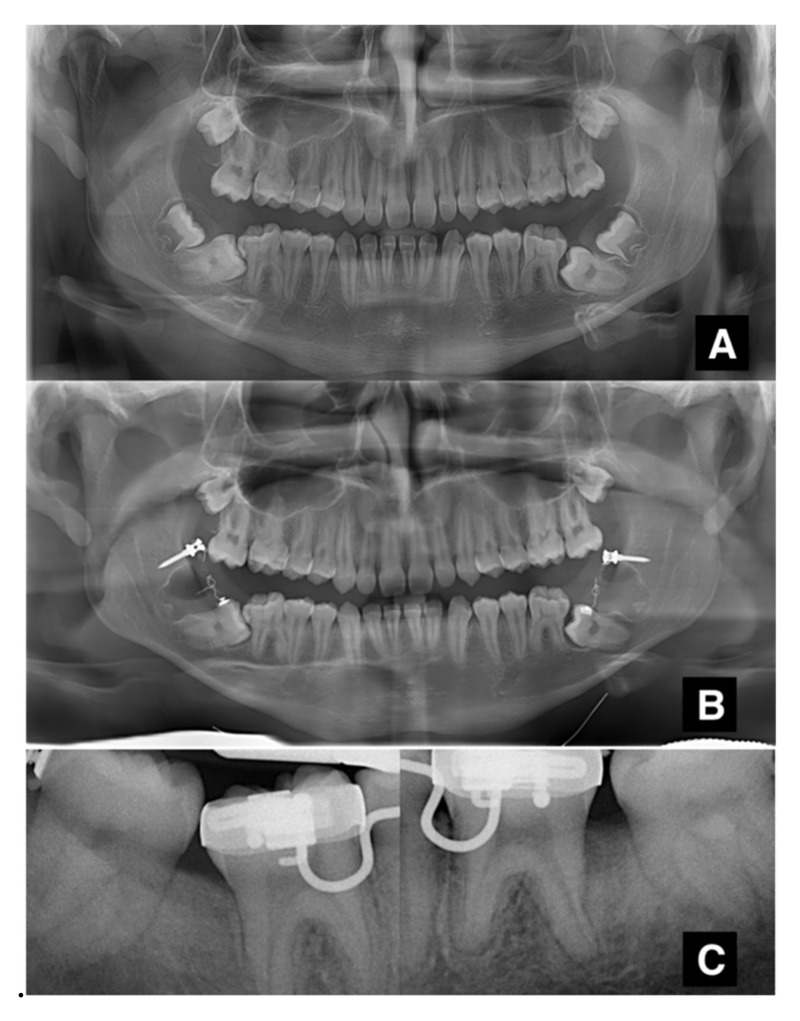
(A–C) Radiographic sequence of a rare bilateral case.
